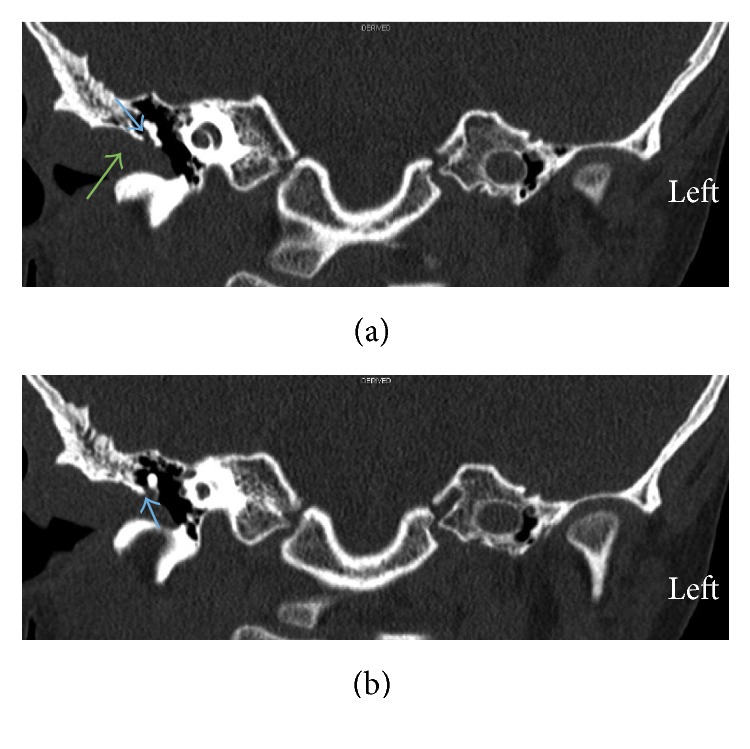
Coronal CT scans. (a) Soft tissue mass in the right external auditory canal (green arrow) extending into Prussak's space (blue arrow). (b) Minimal erosion of the scutum (blue arrow).
